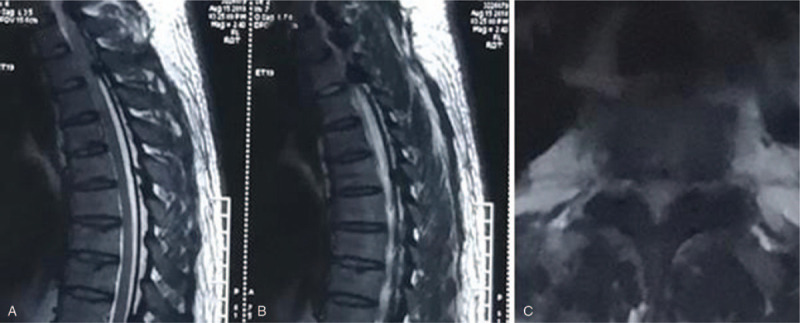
Preoperative magnetic resonance imaging: T2 and 3 ossification of the ligamentum flavum compression of the spinal cord.
